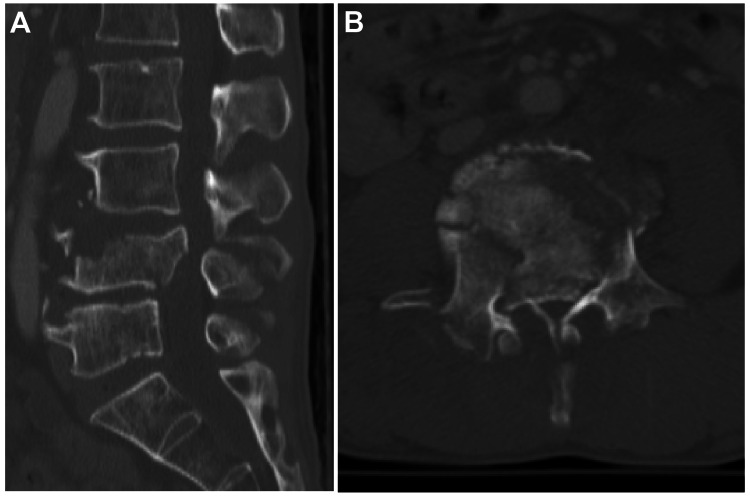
Preoperative CT scan (A: sagittal and B: axial) showed a complete burst fracture of L4. A fractured bone fragment protruded into the spinal canal.
